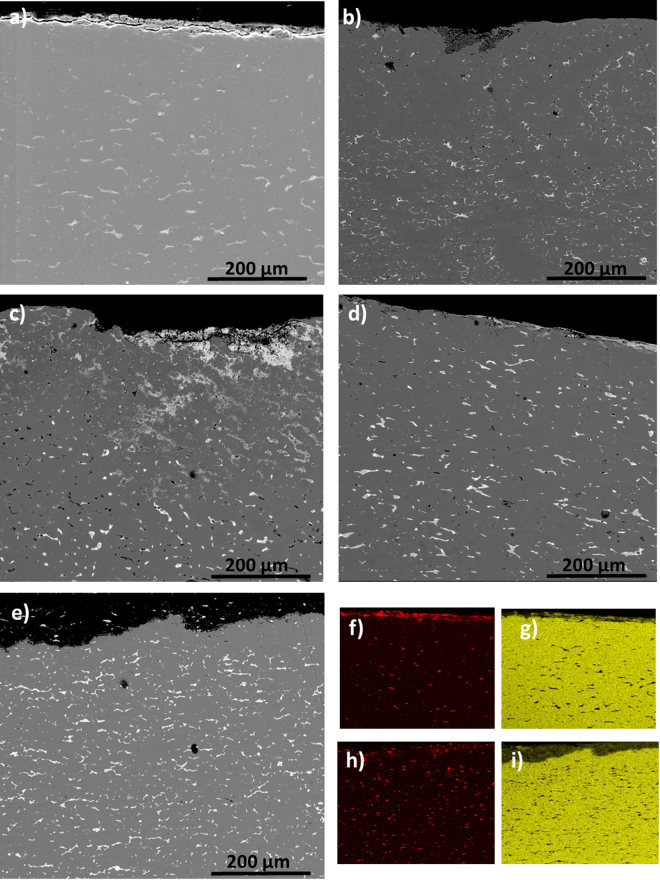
BSE images *of Antoninianus* coins (a) A (b) B (c) D (d) E (e) I and X-ray maps of (f,h) Ag and (g,i) Cu of coins A and I.
